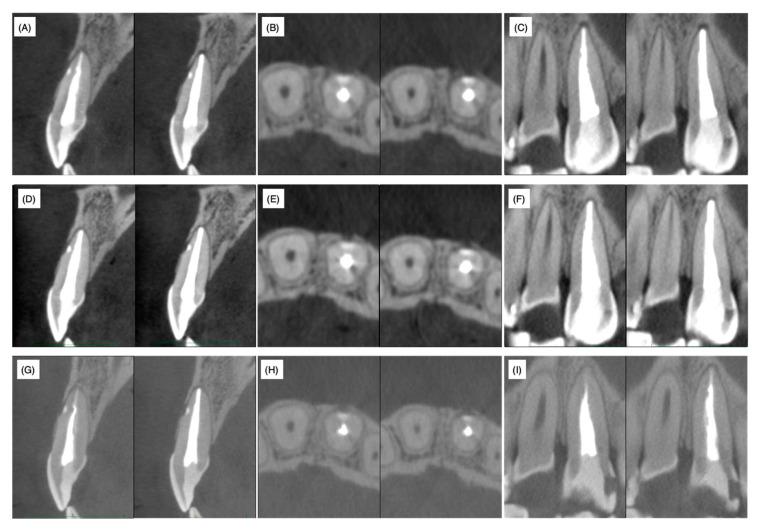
Cone-beam computed tomography images at three months (A) sagittal, (B) horizontal and (C) coronal; one year (D) sagittal, (E) horizontal and (F) coronal and two years (G) sagittal, (H) horizontal and (I) coronal after root filling.
